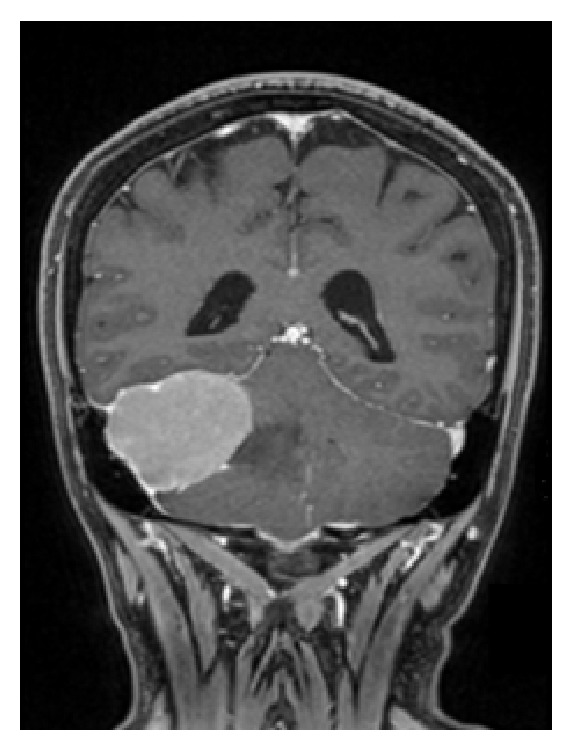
Coronal view of contrast-enhanced MRI scan showing a well-defined tumor with a clear margin. Compression by the mass toward the fourth ventricle caused deformation.
